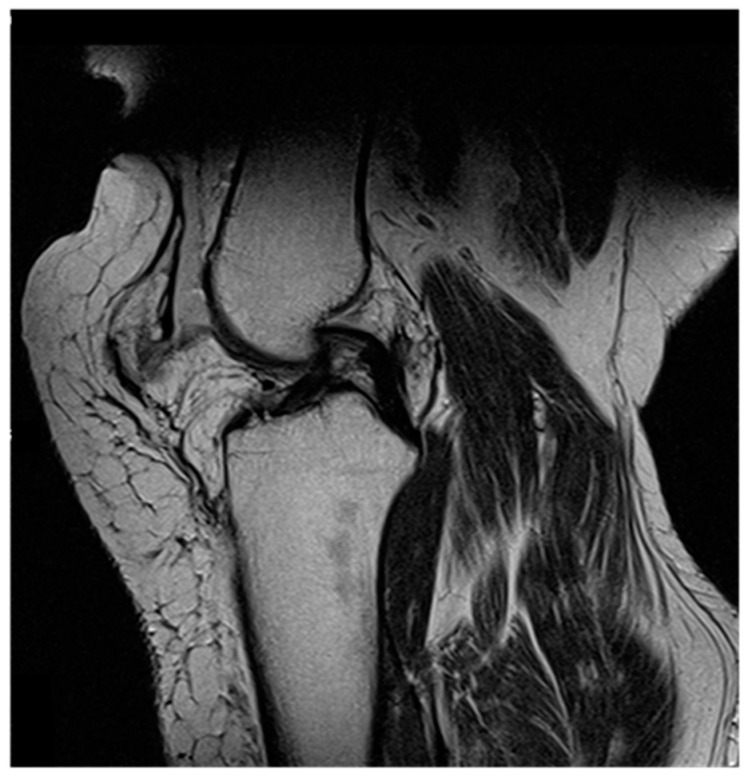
MRI showing overstretching of the knee extensor structures in patient 1.
